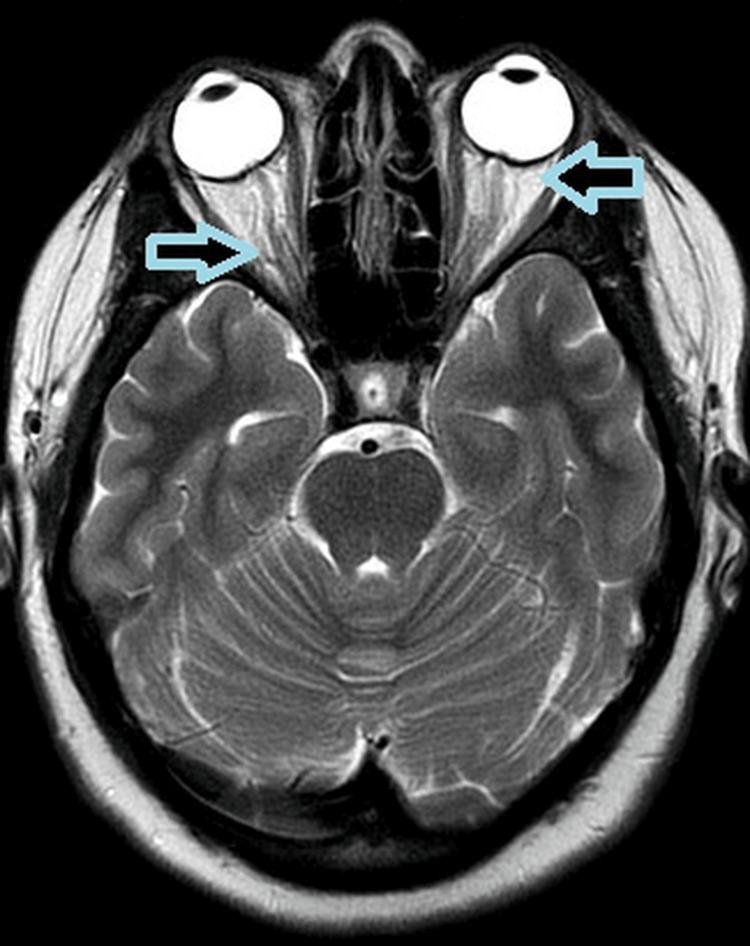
Distension of the bilateral optic nerve sheaths on MRI brain (blue arrows)
